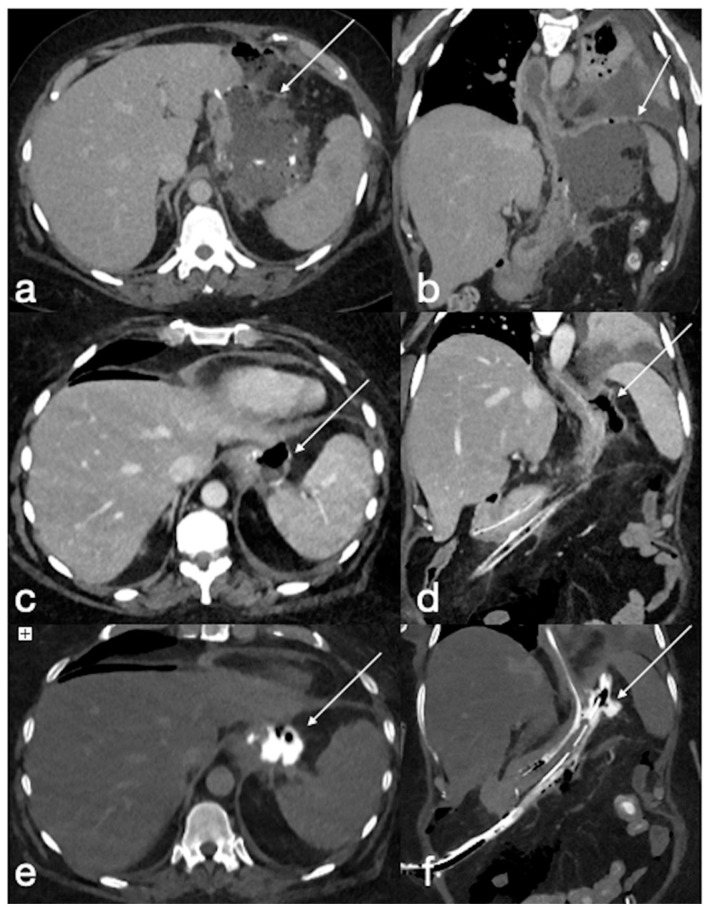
Type III leak after sleeve gastrectomy (a, axial, b, coronal oblique view, arrows) requiring surgical re-suturing. Eight days later, the CT again showed an air–fluid collection adjacent to the proximal gastric suture (c, axial, d, coronal oblique view, arrows) with extraluminal spreading of oral contrast medium (e, axial, f coronal oblique view, arrows). After any attempt at conservative treatment, the patient underwent total gastrectomy.
